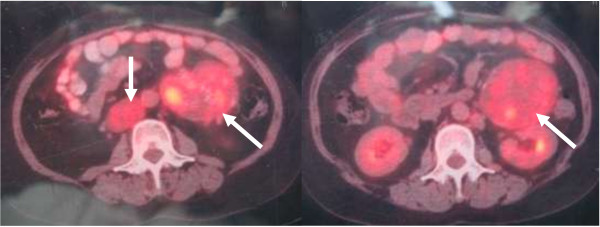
PET/CT revealed abnormally hypermetabolism in the retroperitoneal masses (white arrows) and nodes suggesting metastatic lesions.
